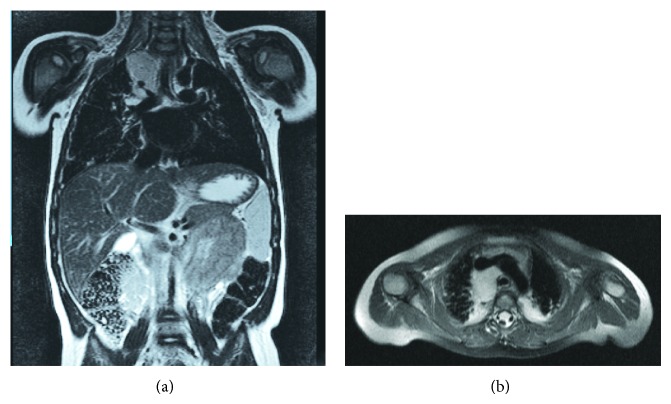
(a) Coronal T2 MRI: complex nodular posterior mediastinal mass infiltrating the right hilum; (b) axial T2 MRI: bilateral mediastinal and hilar lymphadenopathies.
